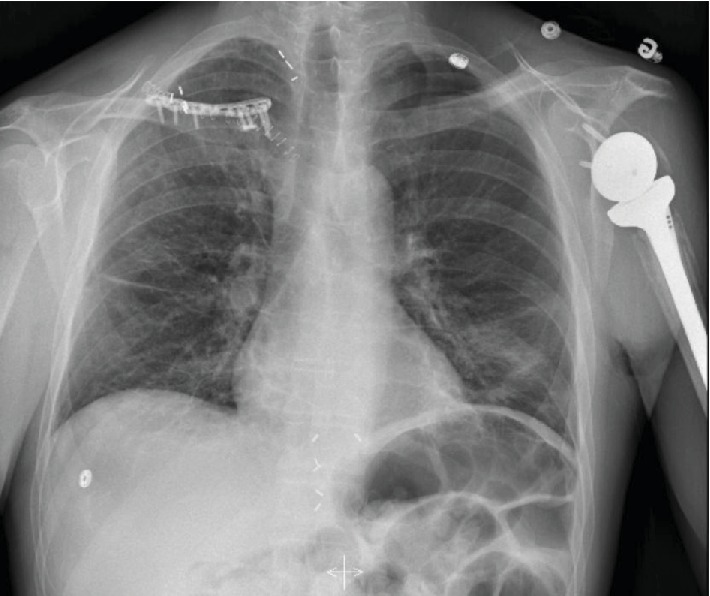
Postoperative chest radiograph following the open reduction and osteosynthesis procedure. The fracture was fixed using an inverted anatomic locking plate.
